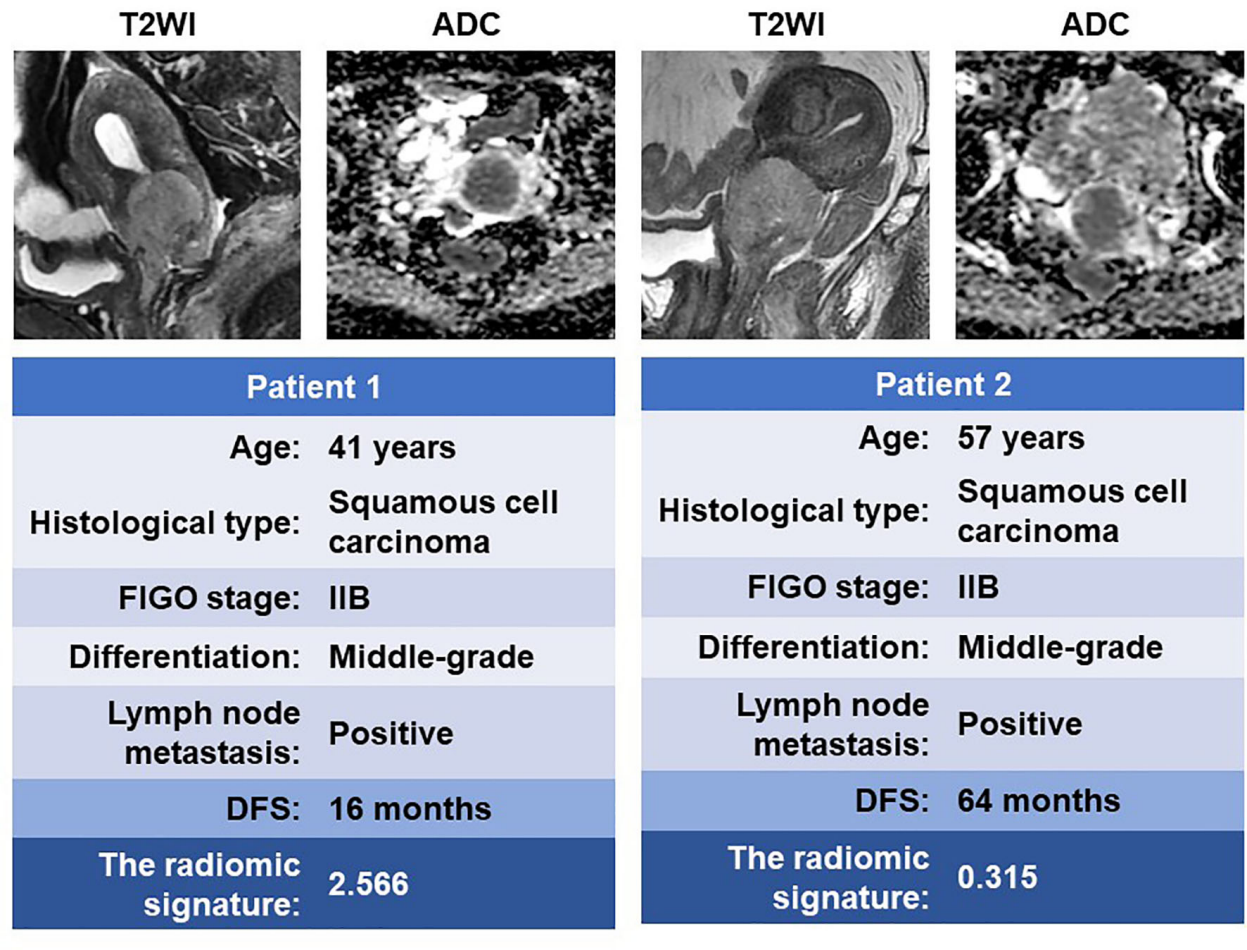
MR images of two patients with similar clinicopathological features but significantly different DFS time. DFS, disease-free survival.
